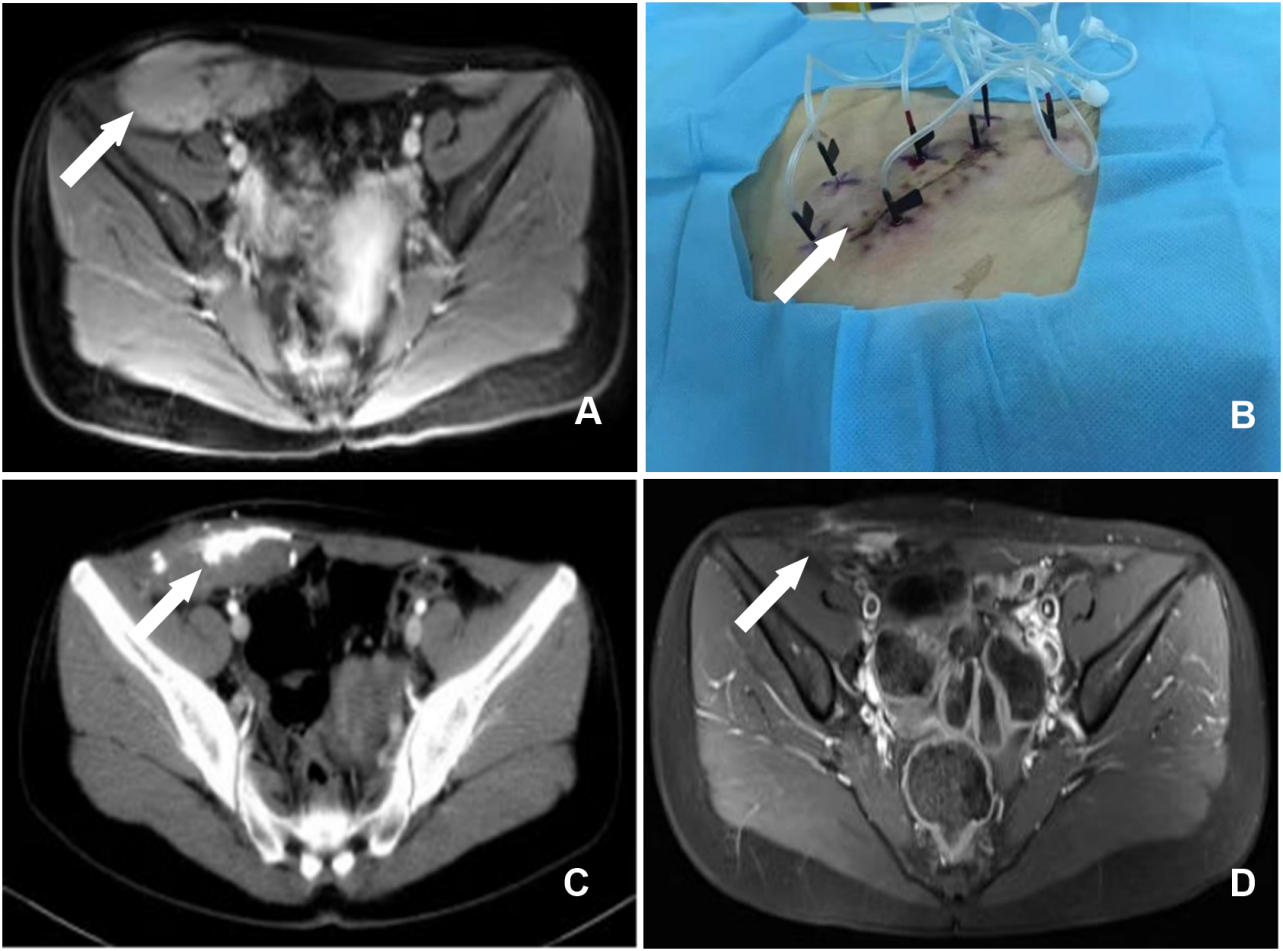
(A) A enhanced MRI examination of the pelvis revealed a massive abnormal signal in the lower right abdomen, occupying the rectus abdominis, transverse abdominis, and internal and external oblique muscles (arrow). (B) Body surface image showing multipoint injections with multiple needles. (C) CT demonstrated the effect of high-density contrast media in the region of the soft tissue mass in the pelvic wall one month after chemical ablation therapy, and the surrounding space was clear (arrow). (D) MRI revealed a considerable reduction in the pelvic wall mass 17 months following chemical ablation therapy and a few increased signals during the enhancement phase (arrow).
